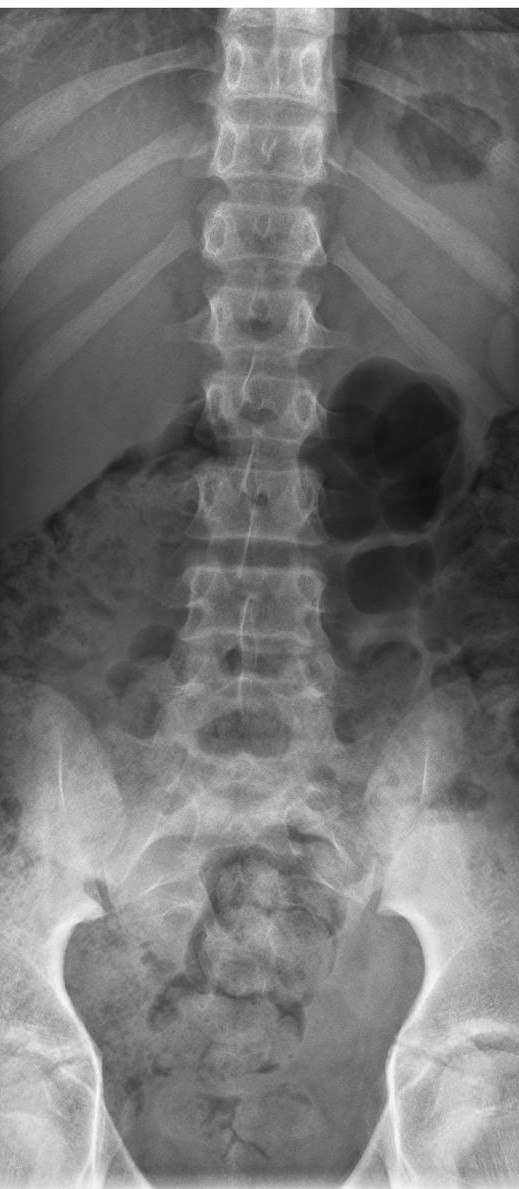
Antero-posterior X-ray lumbar view. Note the absence of scoliosis.
